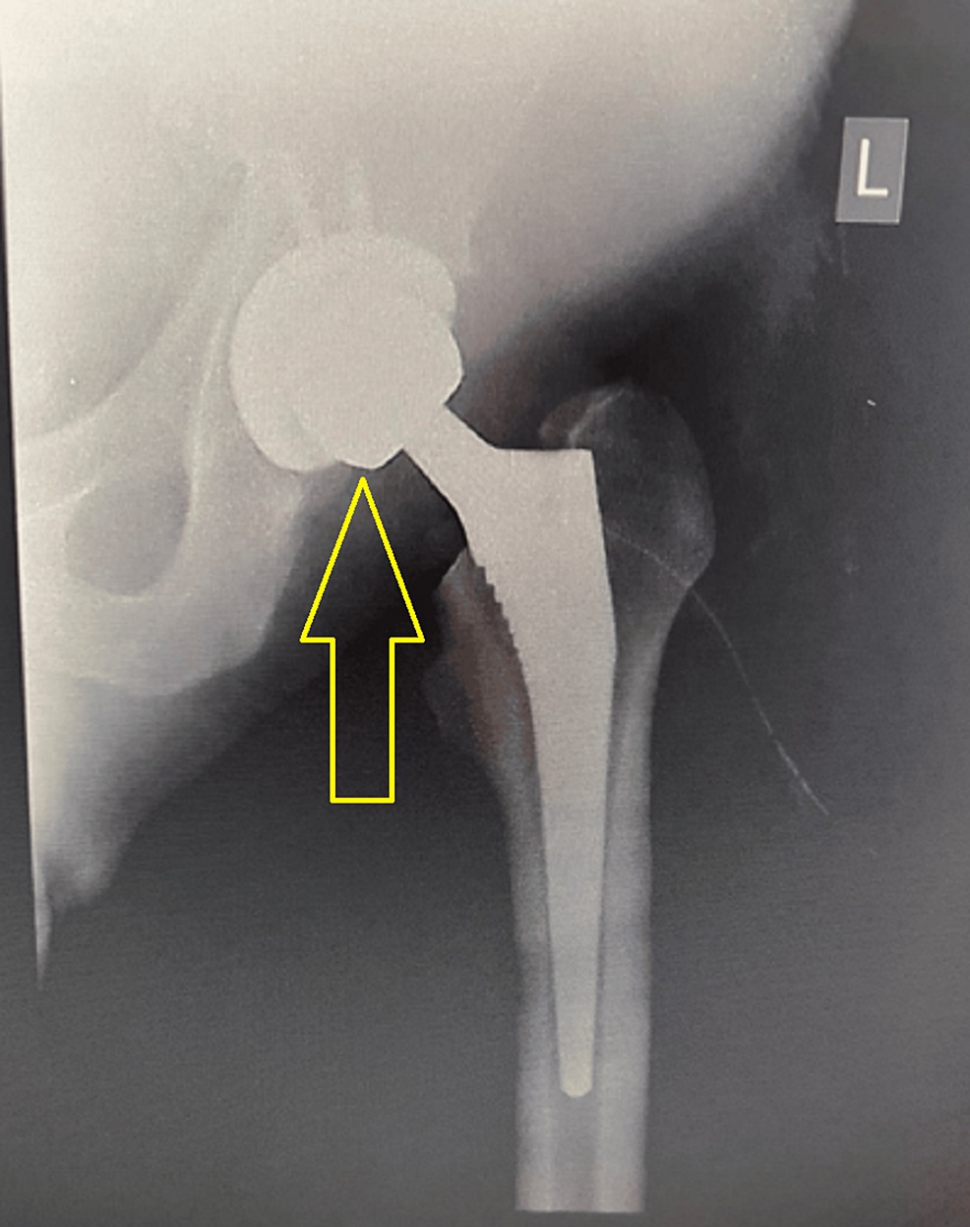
Postoperative X-ray of the left hip joint, coronal plane. X-ray showed successful hip replacement and resolution of symptoms
